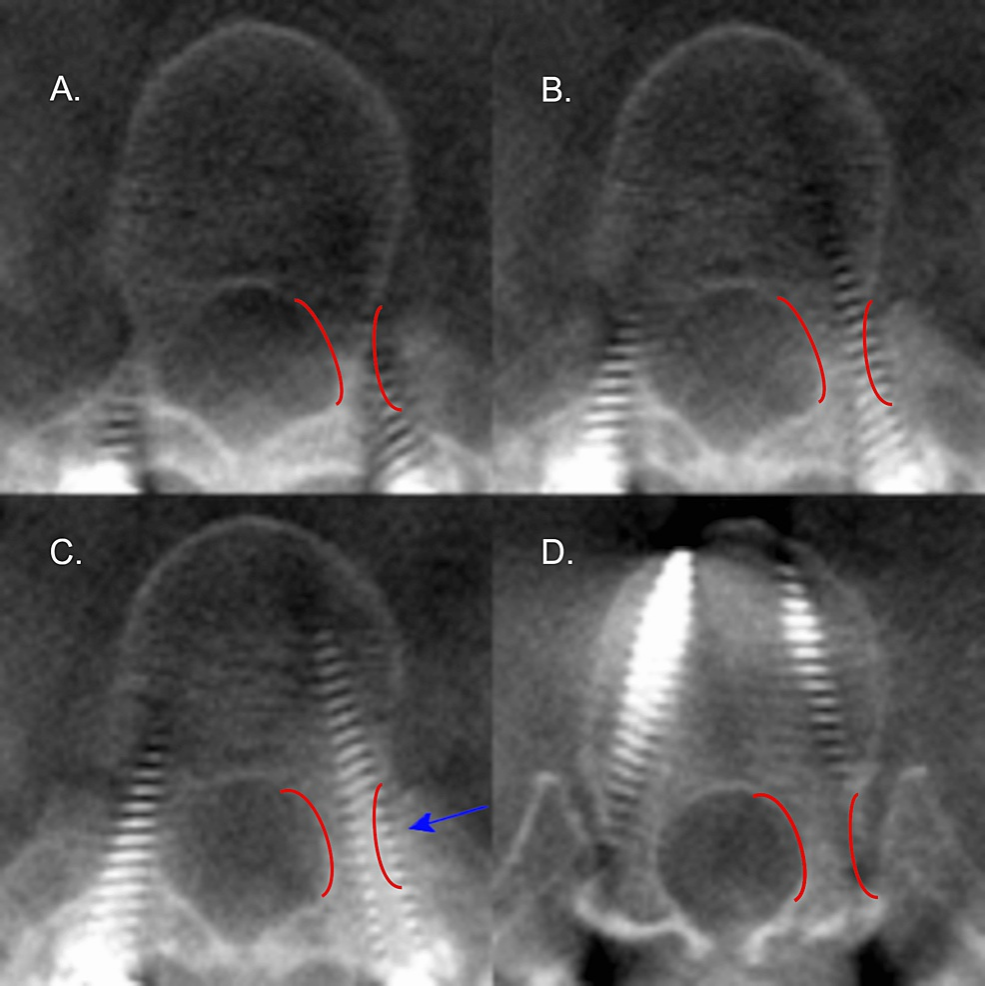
Axial CT radiographs of the left T10 pedicle screw on patient 13. Axial CT radiographs demonstrating the left T10 pedicle screw placement on patient 13 with an in-out-in (IOI) trajectory. Screw placement progresses from A to D. The pedicle of interest is outlined in red. The blue arrow denotes the largest deviation of the screw. Please note all CT images are in radiographic orientation.
